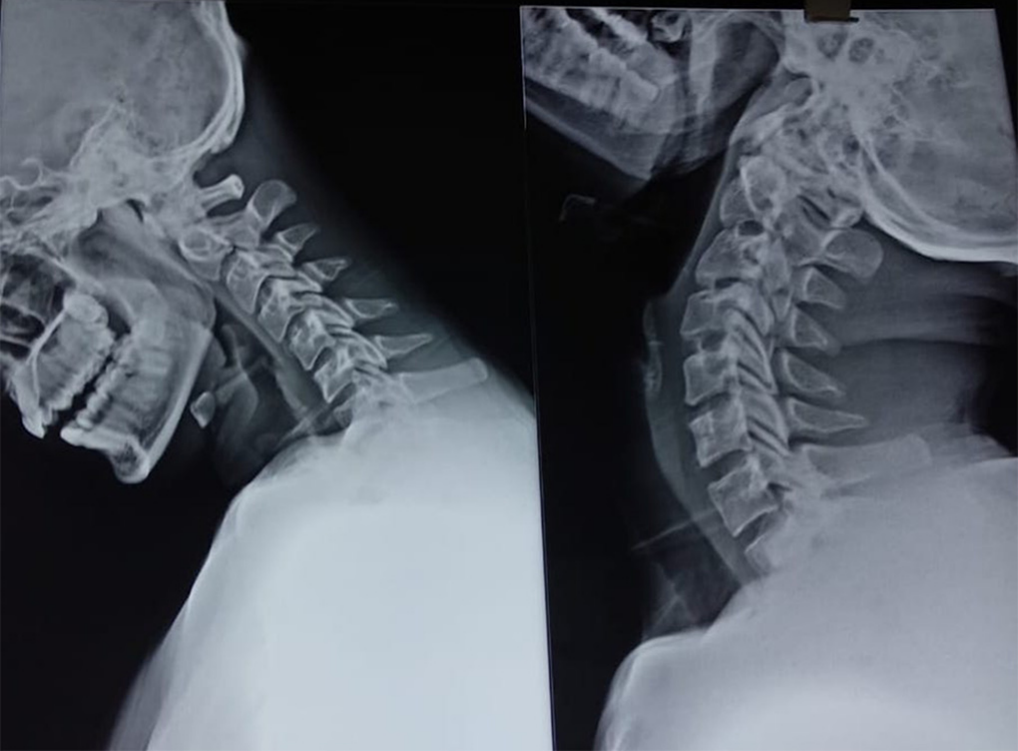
One-year follow-up flexion and extension X-rays show stability and preservation of the motion segment.
